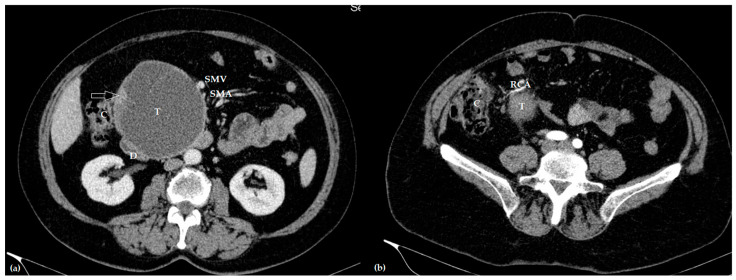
Contrast-enhanced axial computed tomography of the venous (a) and arterial (b) phase showing a large invasive intraductal papillary mucinous neoplasm (T), invading the duodenum (D) and right colic artery (RCA), and in close relationship with the superior mesenteric vein (SMV) and artery (SMA), and ascending colon (C) (the white arrow marks the malignant part of the tumor, without interface with the ascending colon).
